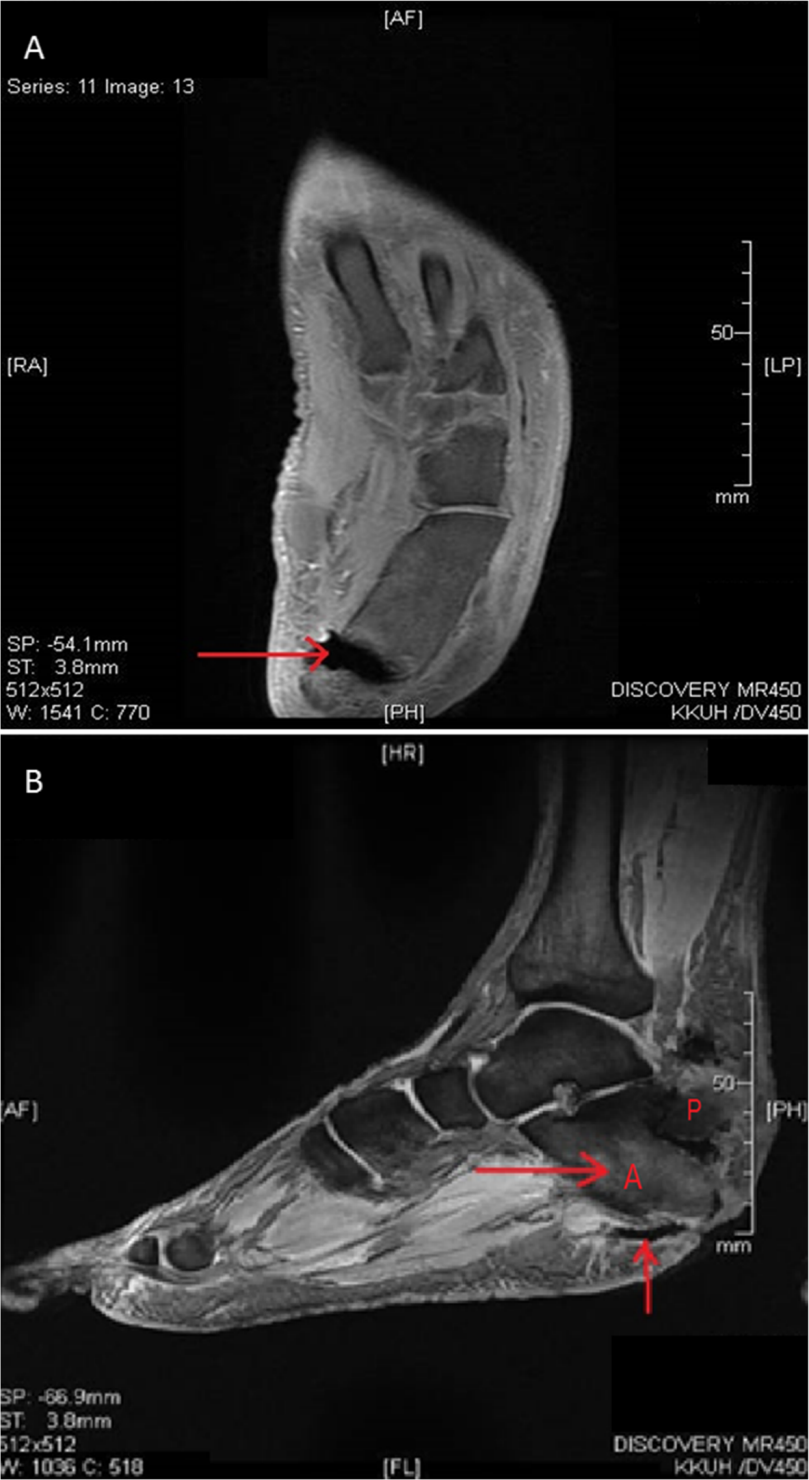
Left foot magnetic resonance imaging. (A) A left foot MRI scan revealed a fracture in the calcaneus. (B) The calcaneus bone is separated into two segments ((A) and (P)) with evidence of anterior segment osteomyelitis and a deep, infected ulcer (arrows).
